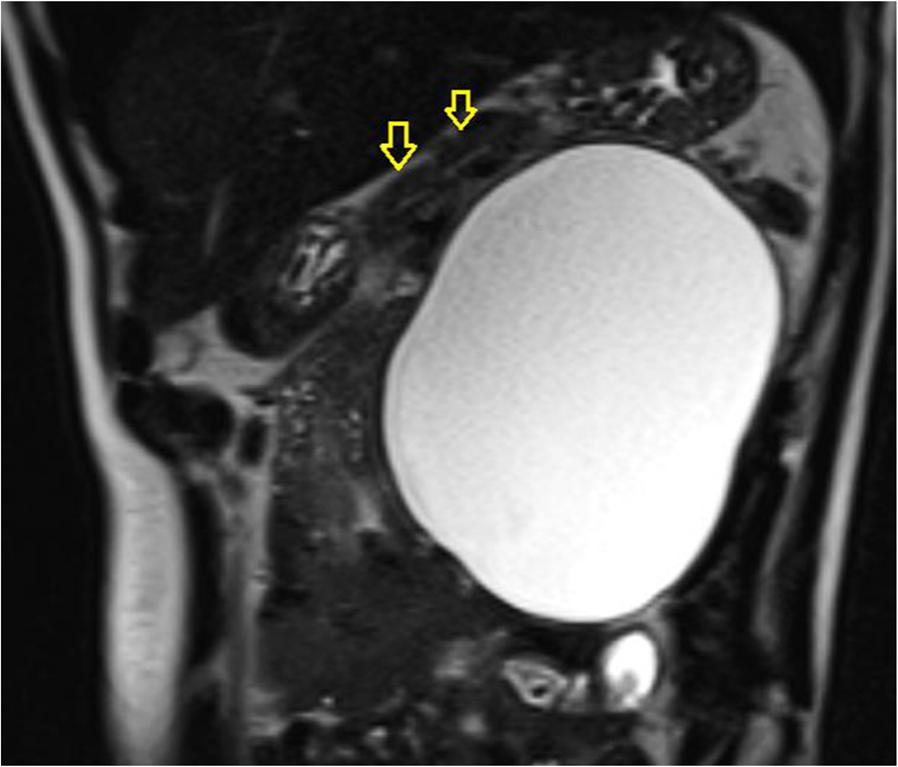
Coronal MRI (T2 phase) showing large retroperitoneal cyst with no septations. The retroperitoneal cyst is separate to the pancreas (Pancreas – yellow arrow).
